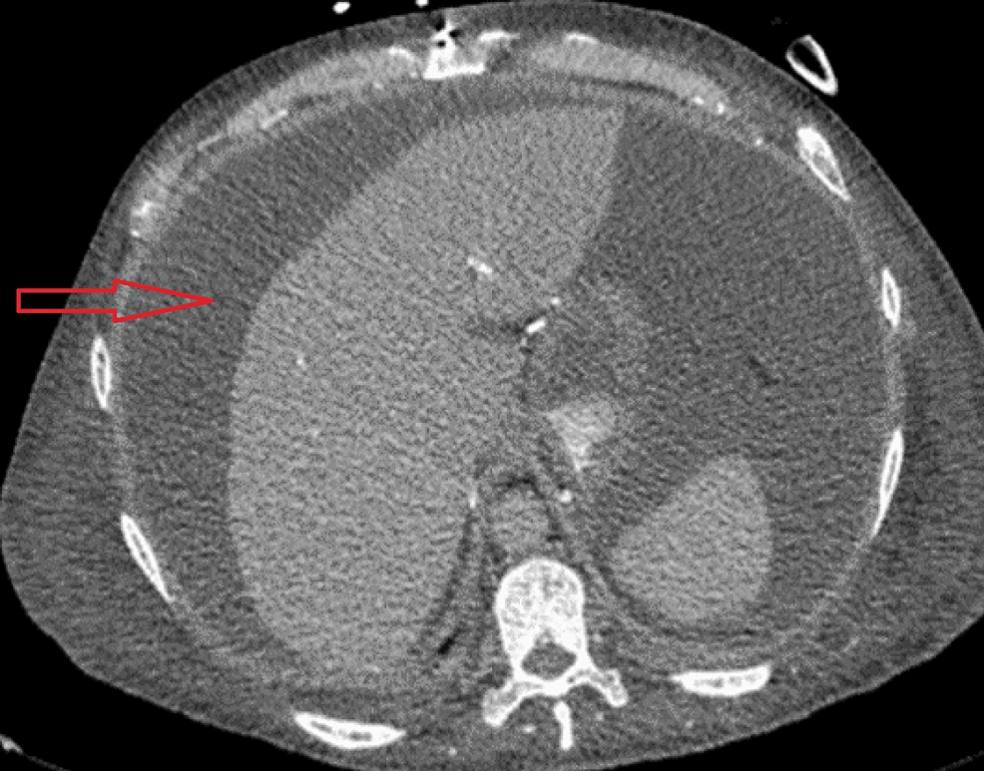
Axial image of contrast-enhanced CT abdomen with massive ascites (horizontal red arrow).
